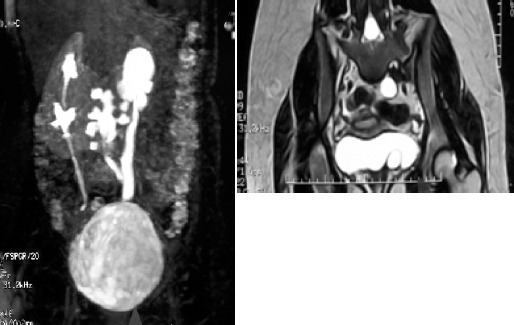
MRI showing bilateral duplex collecting system with double ureter and ureterocoele on the left.
